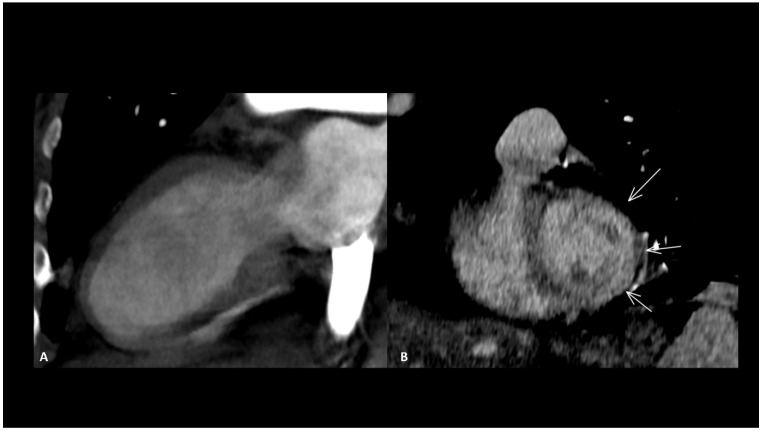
Immune-related Takotsubo disease in a patient treated with anti-PD-1 for recurrent cervical cancer. The patient presented sudden functional impairment with extended akinesia of the mid-cavity and apical wall, with a drop of up to 35% of the ejection fraction. A CT acquired for pulmonary embolism shows apical and mid-cavity ballooning of the left ventricle (A) in the two-chamber view and extended myocardial enhancement of the mid-wall (arrows) (B). Due to the severe clinical compromise, cardiac magnetic resonance was not performed. The diagnosis was obtained after complete recovery of the ventricle function a few days later.
